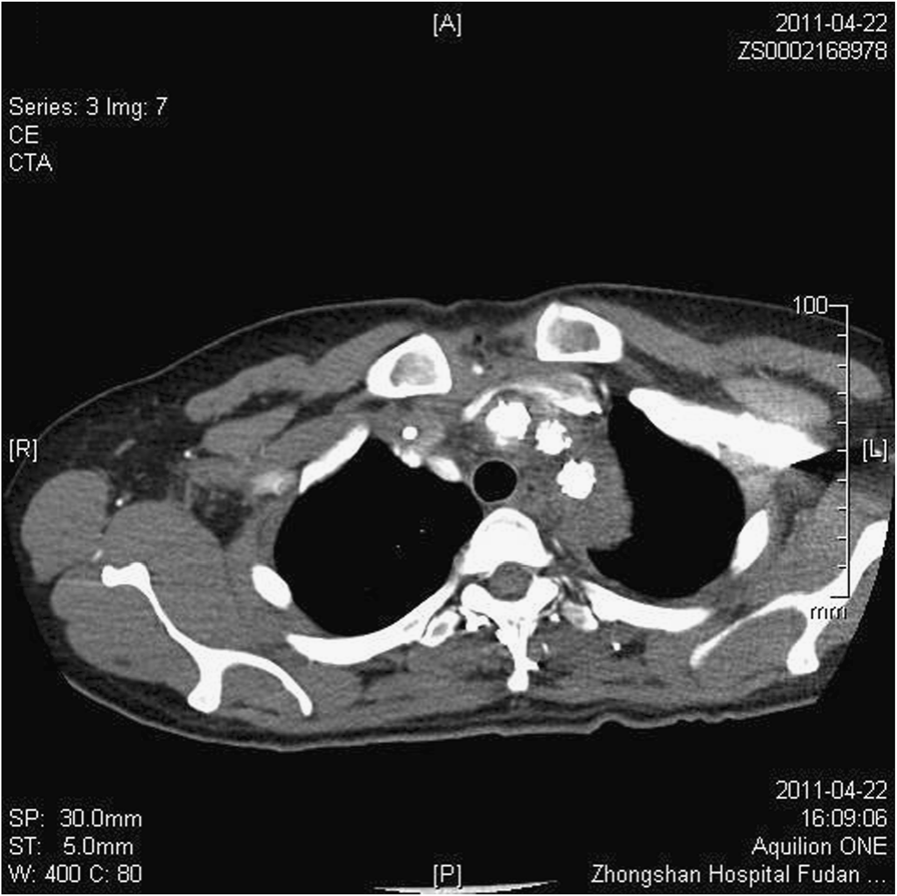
Angio CT Scan shows that all stent grafts were opened and not kinked. No sidearm graft occlusion was found.
